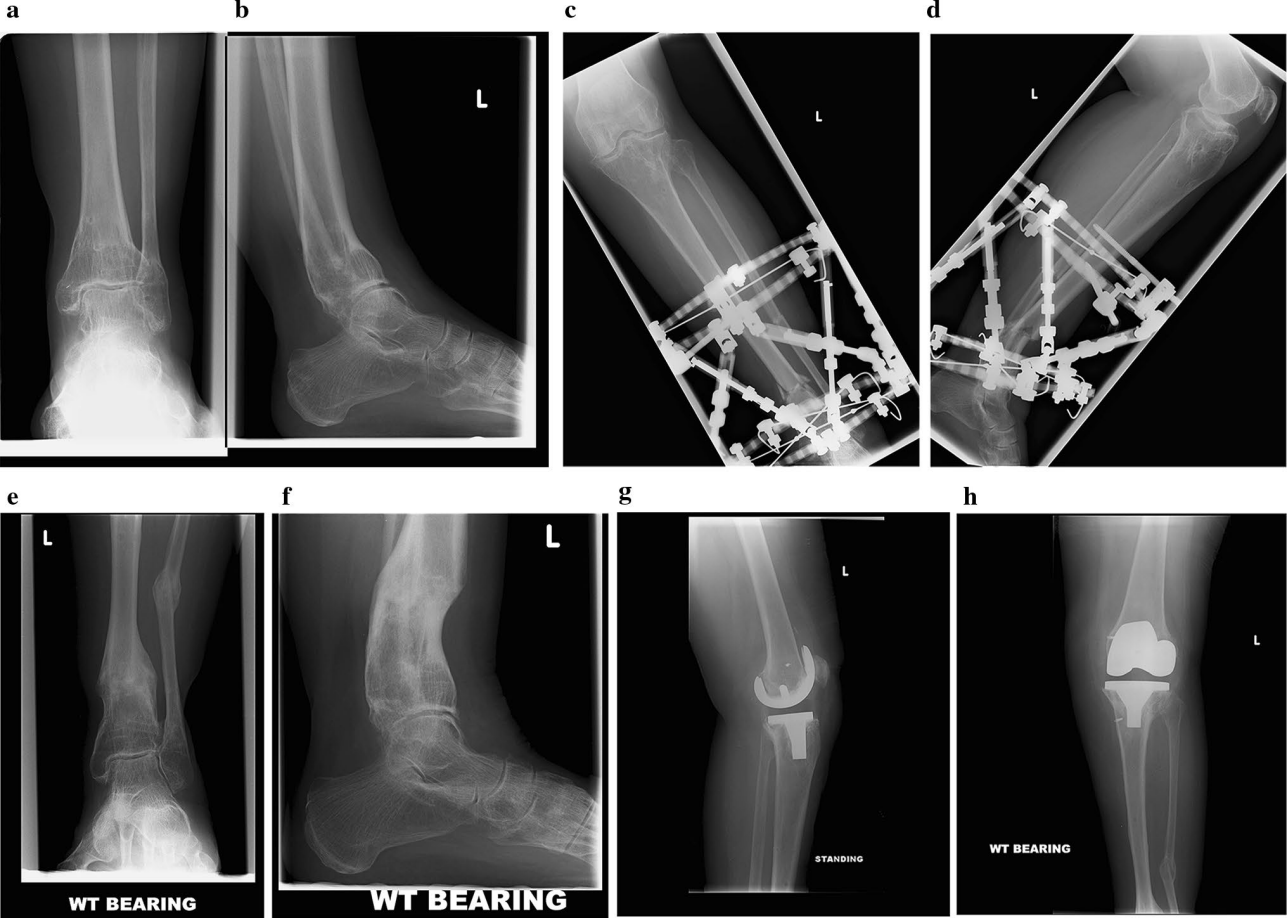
Case 3: a, b Orthogonal radiographs showing supramalleolar tibial deformity. c, d Orthogonal radiographs showing application of Taylor spatial frame and tibial osteotomy for correction of deformity. e, f Orthogonal radiographs showing realignment of mechanical axis with healing of the osteotomy site after removal of frame. g, h Orthogonal radiographs showing total knee replacement in situ performed as a staged procedure
